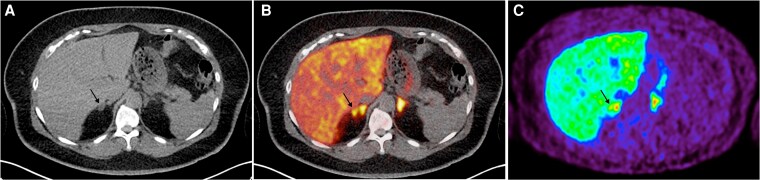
^11^C-MTO PET-CT for case 3—right adrenal nodule seen on CT imaging (A) with low SUVmax over the right medial limb nodule (25.5) compared to the left adrenal gland (28.1) on the fused PET-CT imaging (B) and PET imaging (C). The SUVmax ratio of right adrenal nodule-to-contralateral gland was 0.91 (less than cutoff of 1.25 to define lateralization). Abbreviations: ^11^C-MTO PET-CT, ^11^C-metomidate positron emission tomography-computed tomography; SUVmax, maximum standardized uptake value.
